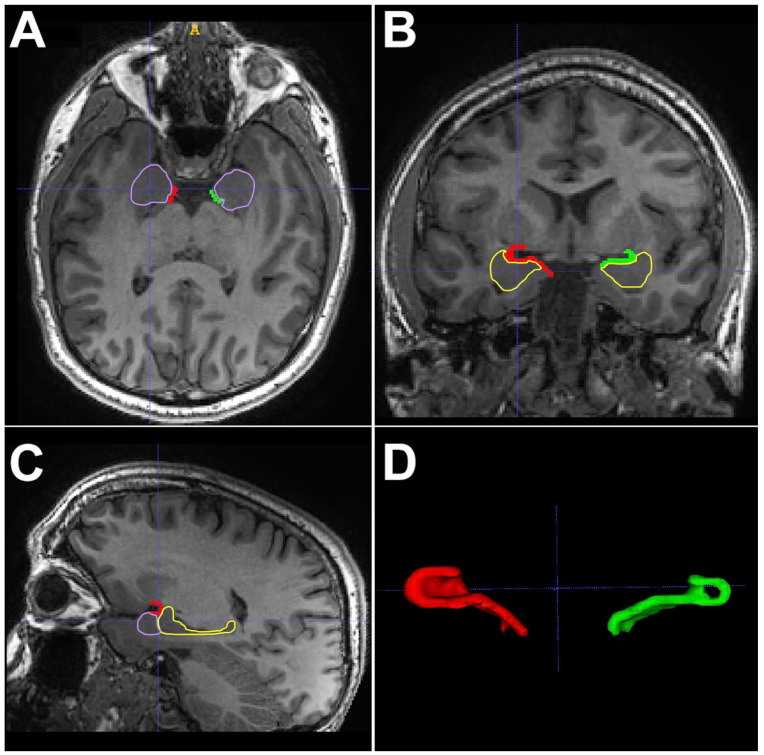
T1-weighted 3D MRI of the brain demonstrating the location of the PC and its relationships with the amygdala (purple) and hippocampus (yellow) in the axial (panel A), coronal (panel B), and sagittal (panel C) planes. Additionally, (panel D) shows a 3D reconstruction of the PC where the classic ribbon shape can be better appreciated.
